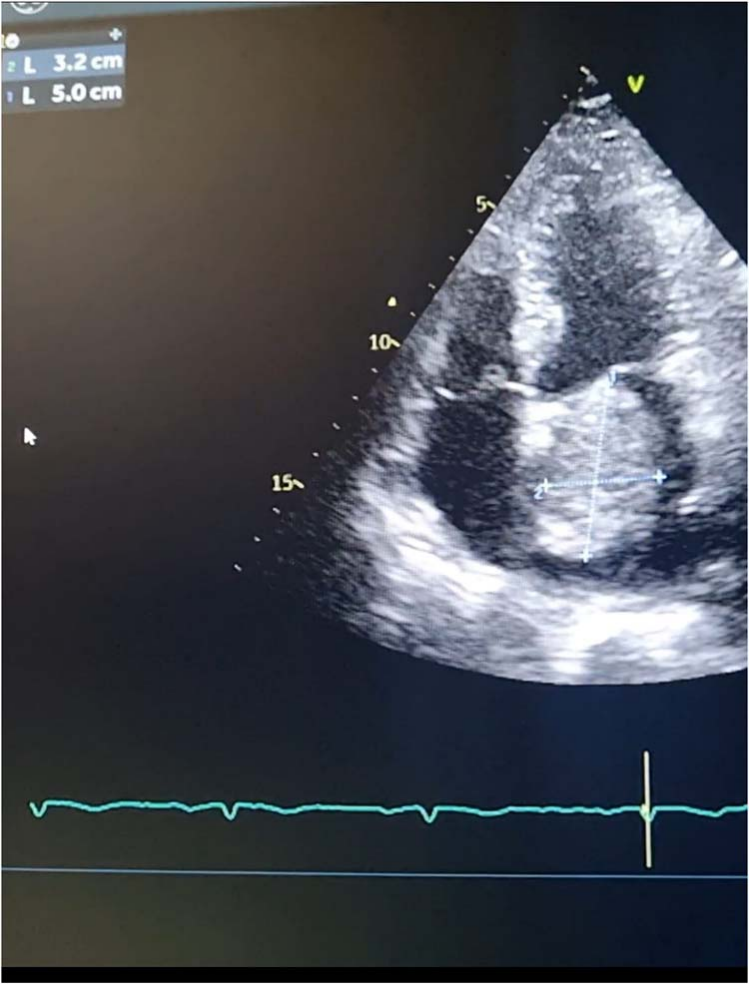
Transthoracic echocardiography. 2D measurements of the LA mass measuring 5 cm ^*^ 3.2 cm.
